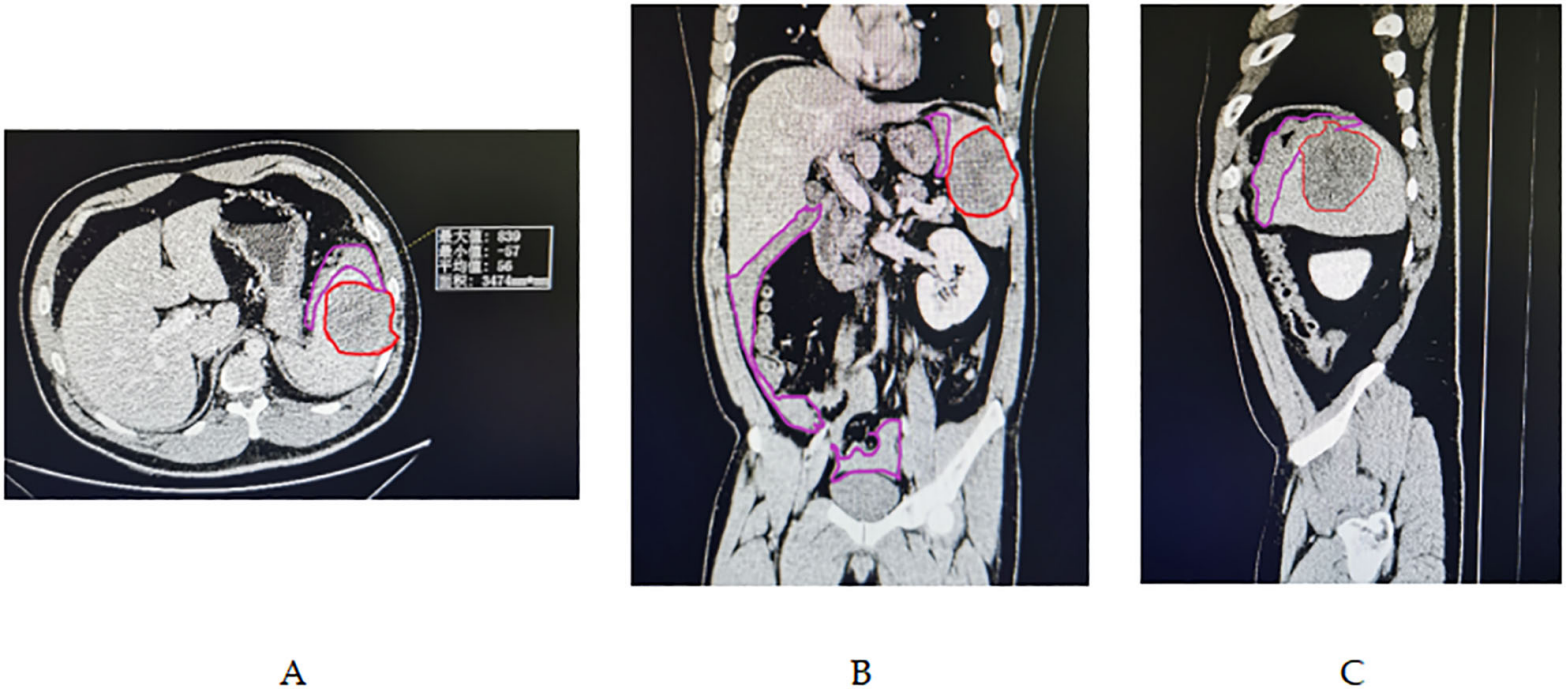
(A-C) Abdominal enhanced CT imaging revealed a large splenic mass, demarcated by the red line, measuring 7.8 x 6.5 cm in diameter, situated within a homogeneous low-density area. This mass was well-separated from the surrounding healthy splenic tissue. Additionally, a substantial amount of hemoperitoneum was detected within the pelvic, perisplenic, and perihepatic regions, as indicated by the purple line demarcating the area.
